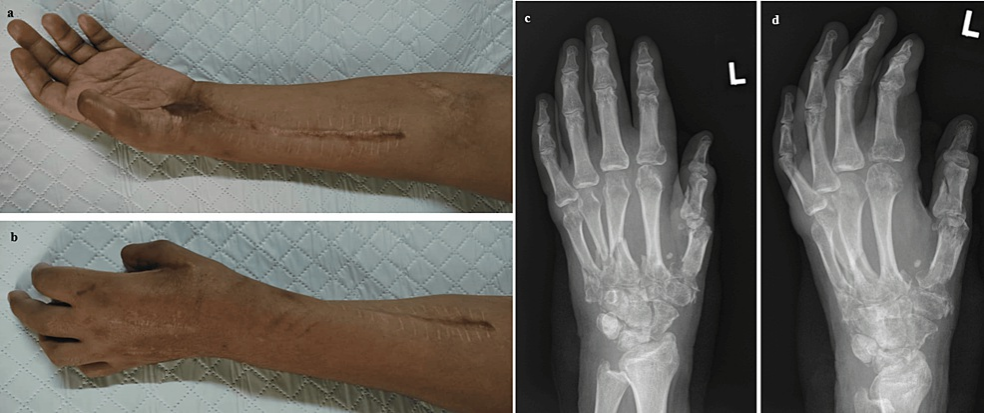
Clinical photos and left-hand radiographs at six months reveal significant improvement and positive progress in the patient's recovery journey. a-b) Clinical pictures reveal a fully healed wound on the left forearm, with the full-thickness skin graft applied over the left wrist flexor crease and thenar eminence displaying successful integration, all without any signs of contracture. c-d) Left-hand radiographs at six months show fracture callus formation with satisfactory alignment.
